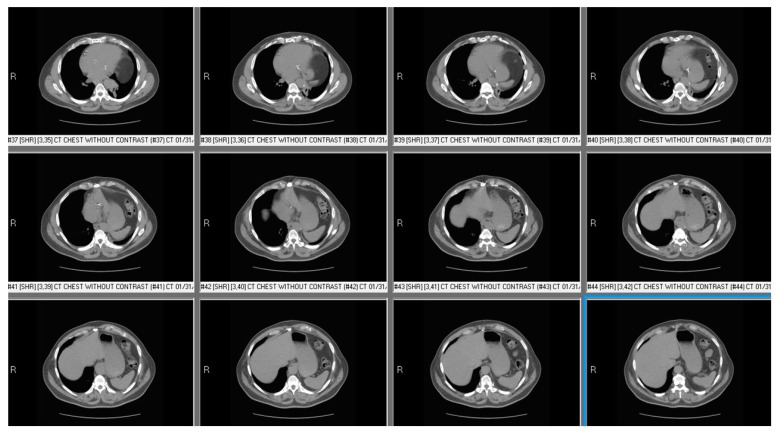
Computed Tomography (CT) chest of patient with left diaphragmatic palsy showing abdominal contents beside heart and at higher level compared to liver suggesting left diaphragmatic palsy.
